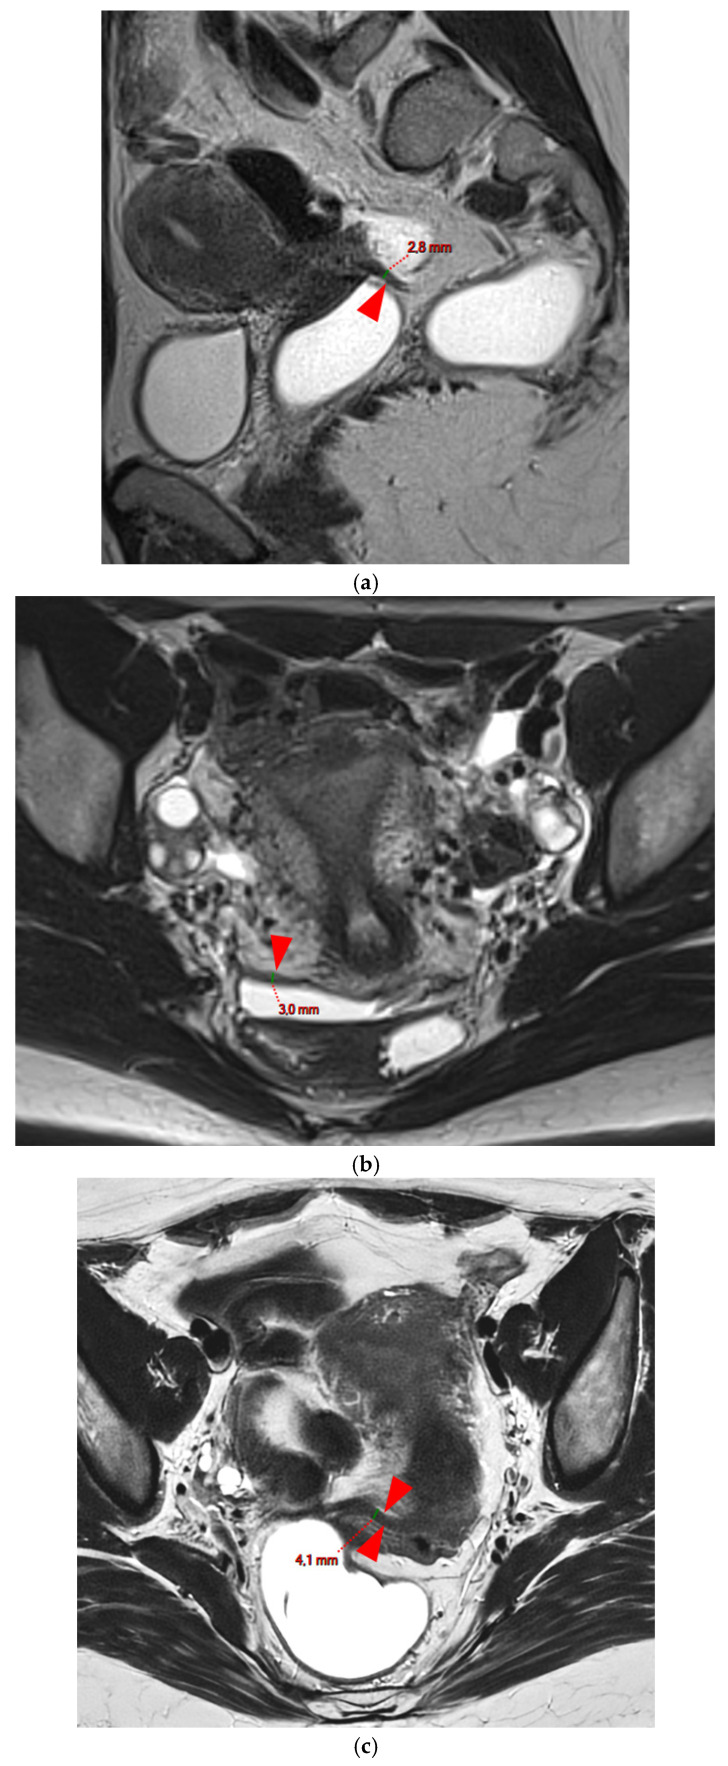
Pelvic MRI scans of three patients with thick (>2 mm), smooth, and regular USLs (HTD type 3A). (a) Sagittal T2WI: a thick (2.8 mm), smooth, tapering-shaped left USL (*arrowhead*). (b) Axial T2WI: regularly thickened (3 mm) right USL with a smooth surface (*arrowhead*). (c) Axial T2WI: regularly thickened (4.1 mm) right USL with a smooth surface (*arrowheads*).
